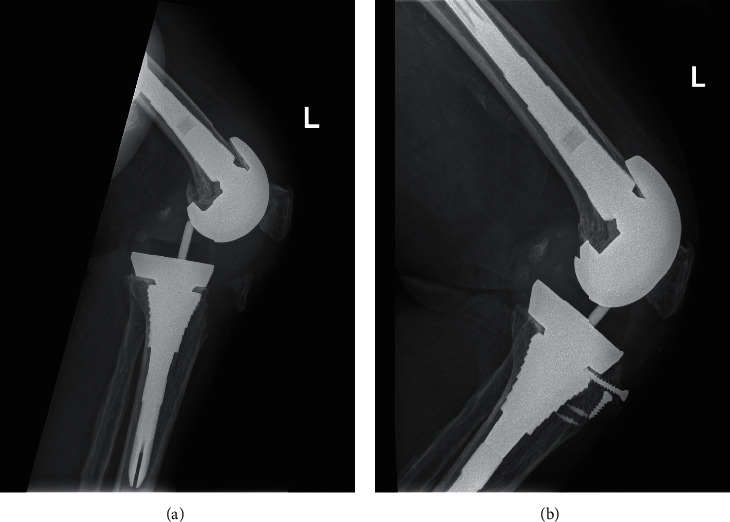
(a) Lateral radiograph of the left knee showing the posttraumatic tibial tuberosity avulsion fracture and the subluxated prosthesis. (b) Lateral radiograph of the same knee at the most recent 2-year follow-up showing a healed fracture.
